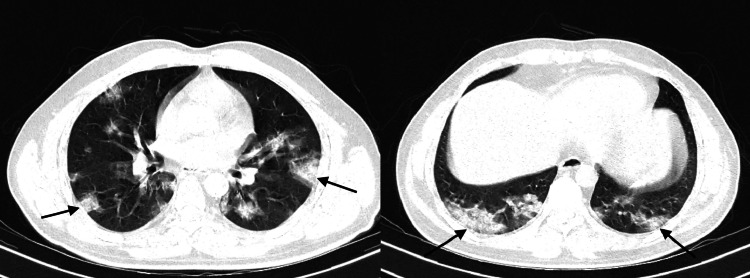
Axial CT scan of the chest with lung window in the same patient after two weeks. The arrows show extensive reduction in ground-glass opacities involving the bilateral lower lobes.
